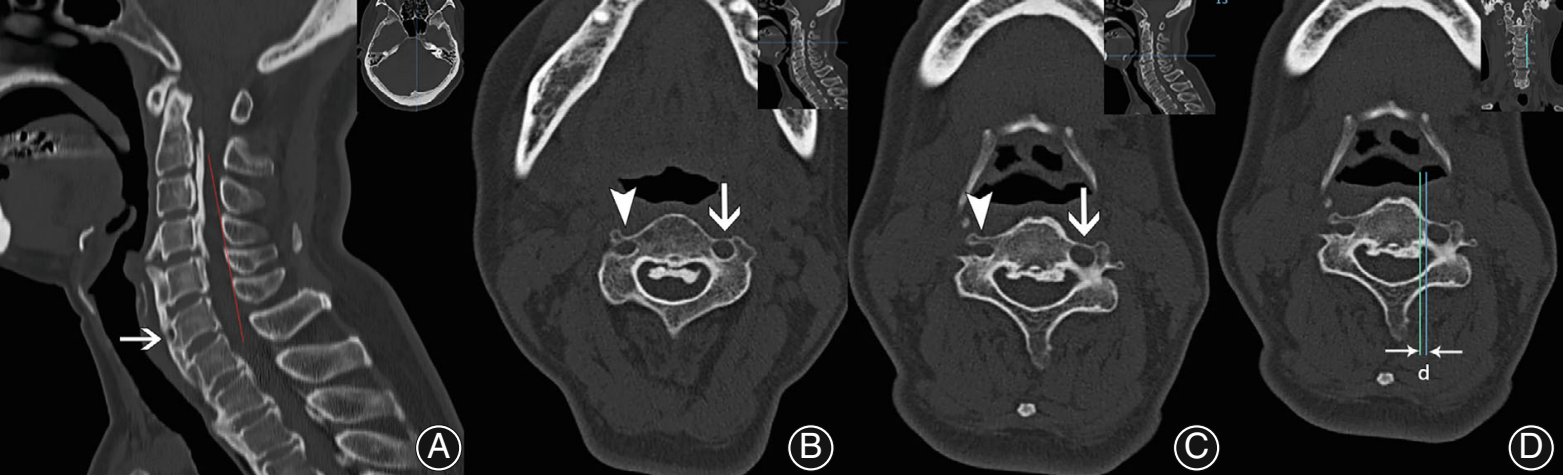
Cervical CT scan demonstrates a mixed OPLL from C2–C6. (A) Sagittal reconstruction CT. (B, C) Axial CT cuts of C3 and C4. The K‐line is positive (A, red line), with evident anterior multi‐segmental calcification (A, arrow) that is indicative of DISH. The ossific ligament, occupying 1/3 of the cervical spine canal in some segments, has a broad base attached to the posterior edge of the vertebral bodies (B, C). The transverse CT scans show the morphological difference between the left and right transverse foramina at C3 (B, right, arrowhead; left, arrow) and C4 (C, right, arrowhead; left, arrow: medial erosion of the vertebrae due to artery tortuosity can be observed). The left transverse foramina of C4 is significantly bigger and more closed to the ipsilateral uncinate process tip than the right side (D, the blue line indicates the border of the internal cortex of transverse foramina of C4 in the transverse cut; the green line indicates the ipsilateral uncinate process tip; the distance (d) between the two lines is 1.1 mm). DISH, diffuse idiopathic skeletal hyperostosis
